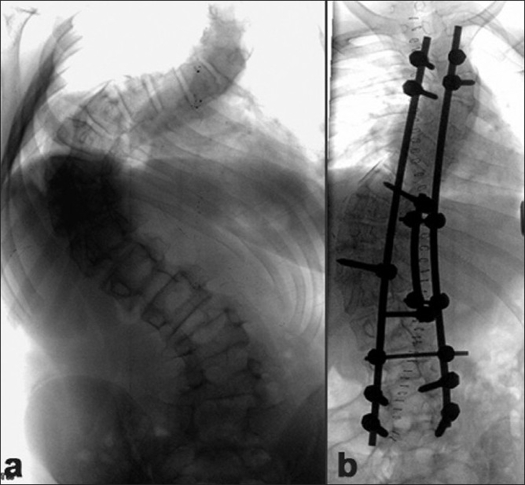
(a) Pre-operative X-ray of dorsal, lumbar spine including lumbosacral junction (anteroposterior view) of a 15 year old female with rigid Lenke Type 4C curve having a major Cobb's angle is 104° (b) post-operative radiograph showing correction achieved with use of a three rod technique. Postoperative major Cobb's is 48°
